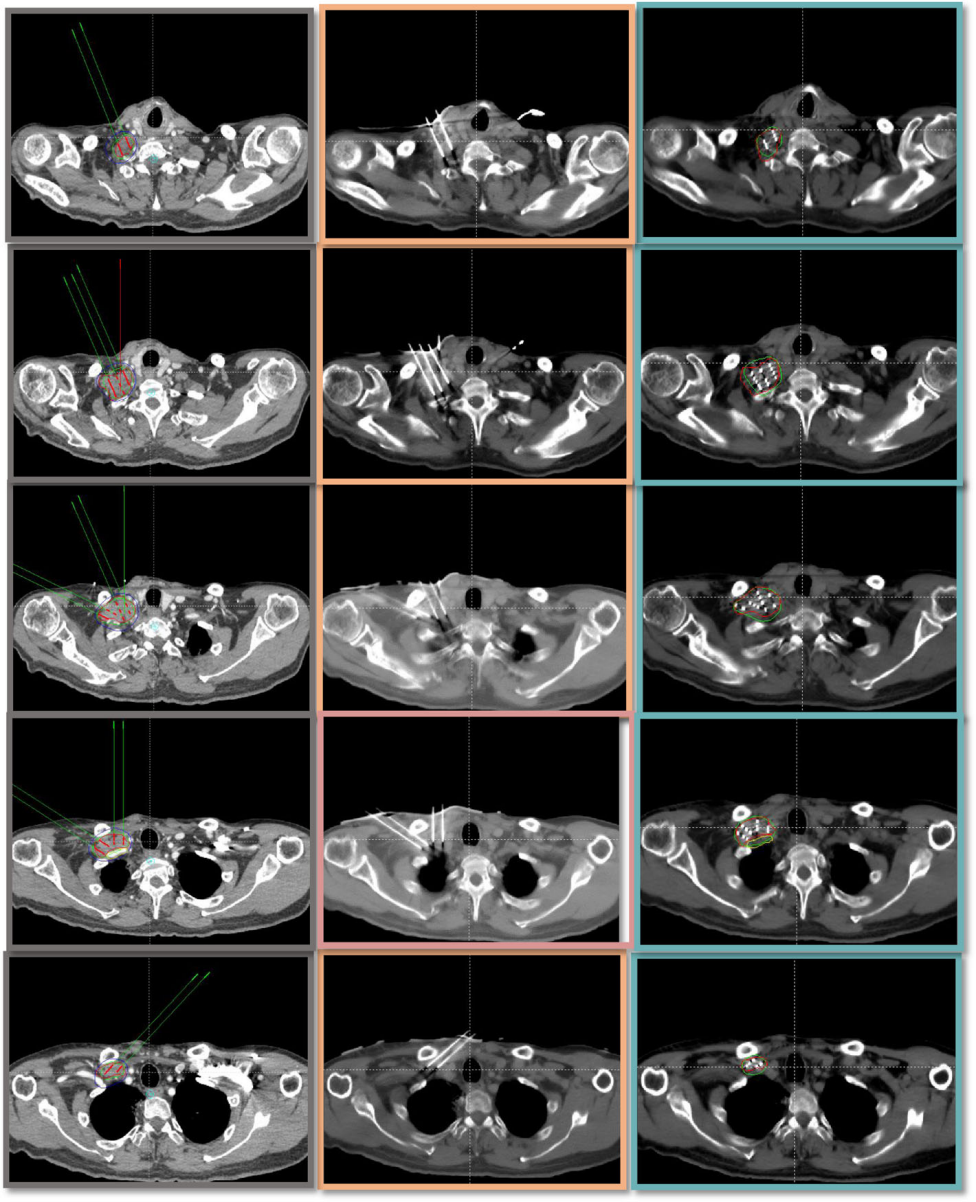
The CT tomographic images of the preimplant and postimplant plan. (Squamous carcinoma of the esophagus yT2N1M1b. Stage IV. Right clavicular lymph node metastasis, EBRT:DT60Gy before). The first column lists all the CT tomographic images of the preimplant plan. The second column shows locations of needles before implantation the RIS. The third column lists the images of the postimplant plan in which includes seeds positions, dosimetric evaluations of GTV and adjacent OARs
